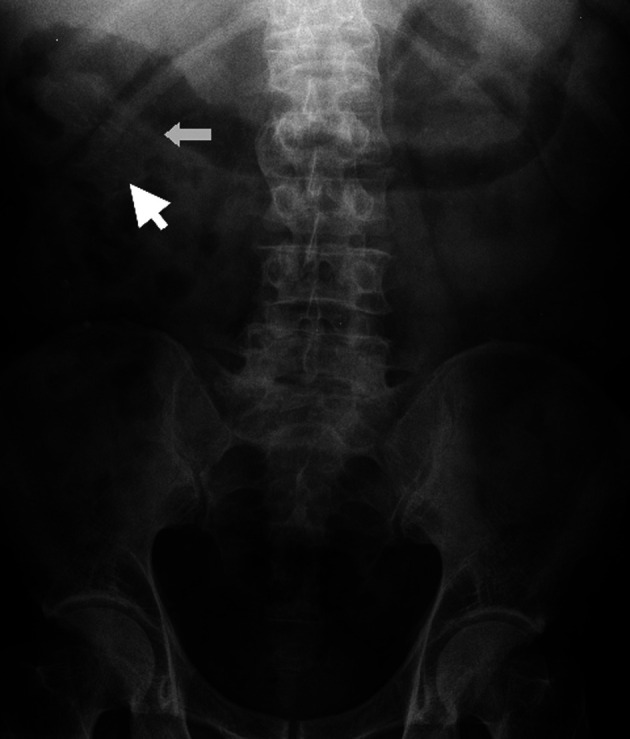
Abdominal plain radiography demonstrating multiple linear and thread-like calcifications at the site of the ascending colon (arrow).
